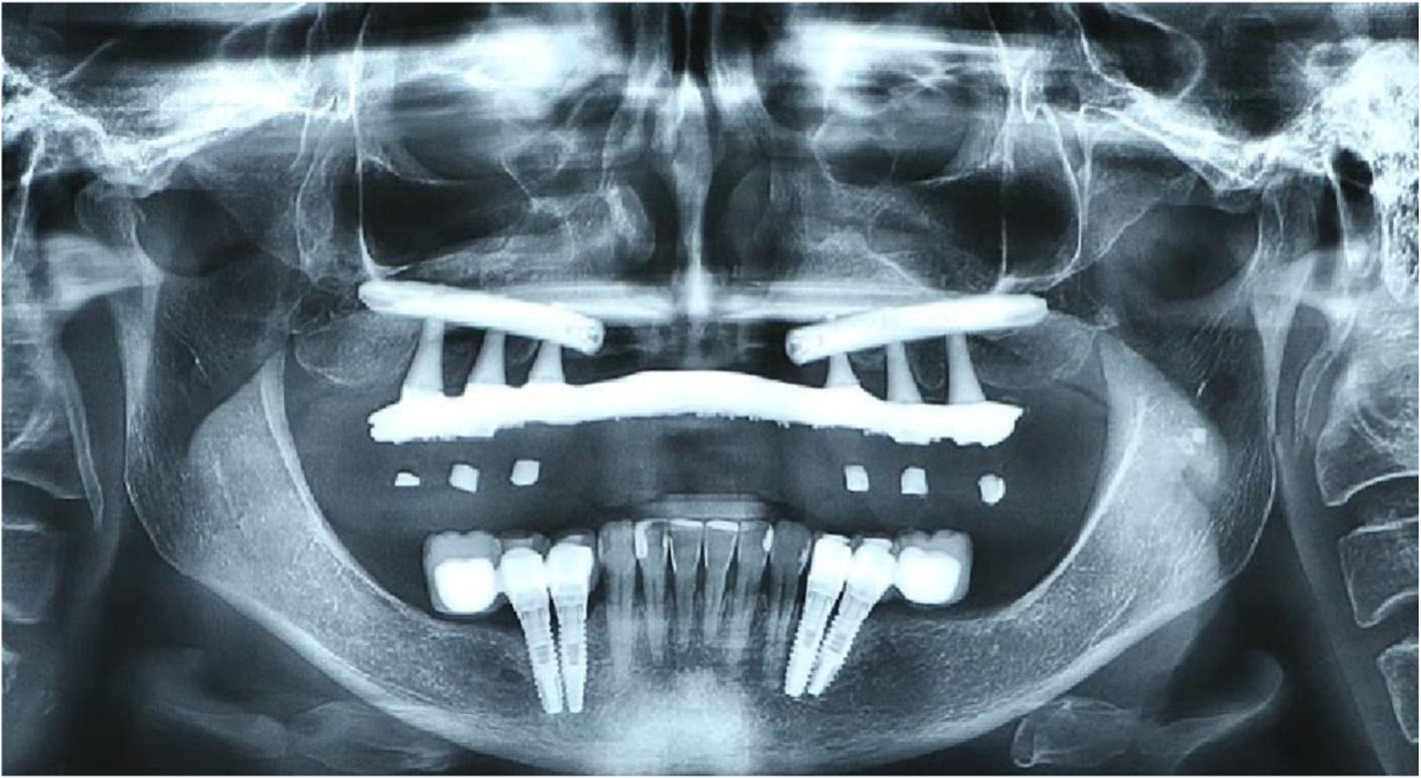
Panoramic radiography of the follow-up
